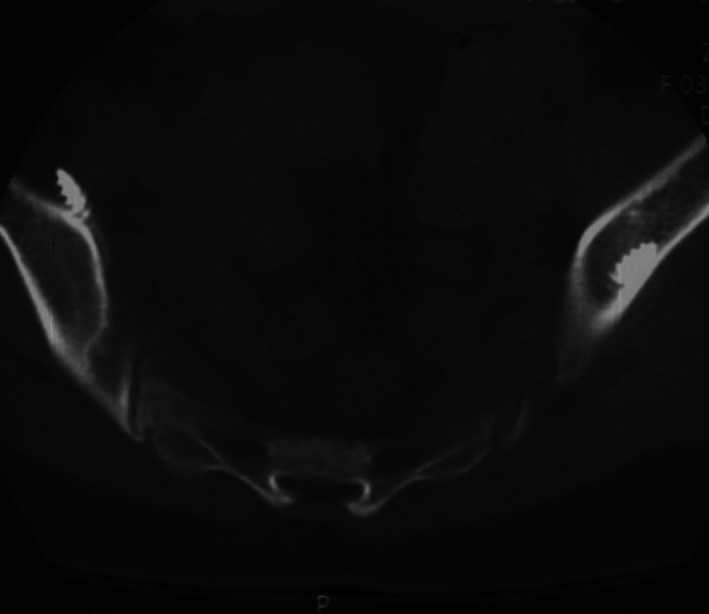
Postoperative computed tomographic scan on axial slice showed an anterior breach of S2AI screw on the right side
